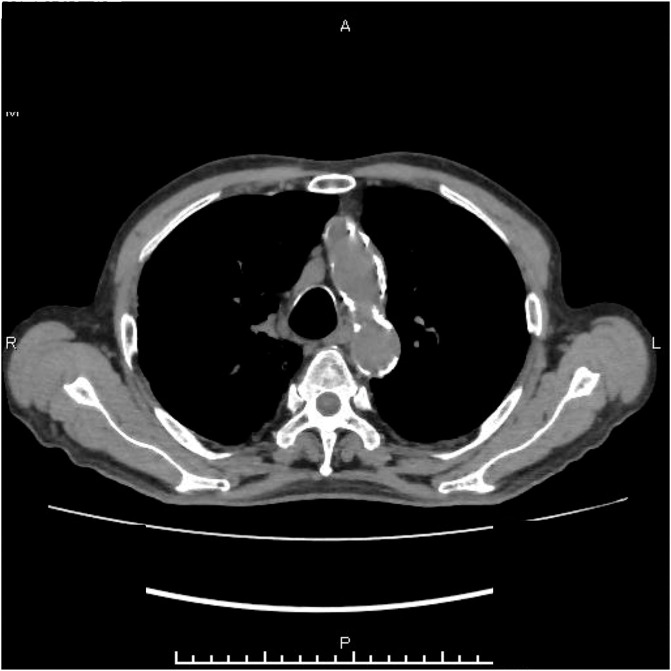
Fig. 1 Computed tomography at the time of admission did not reveal any particular findings suggestive of an inflammatory focus.
